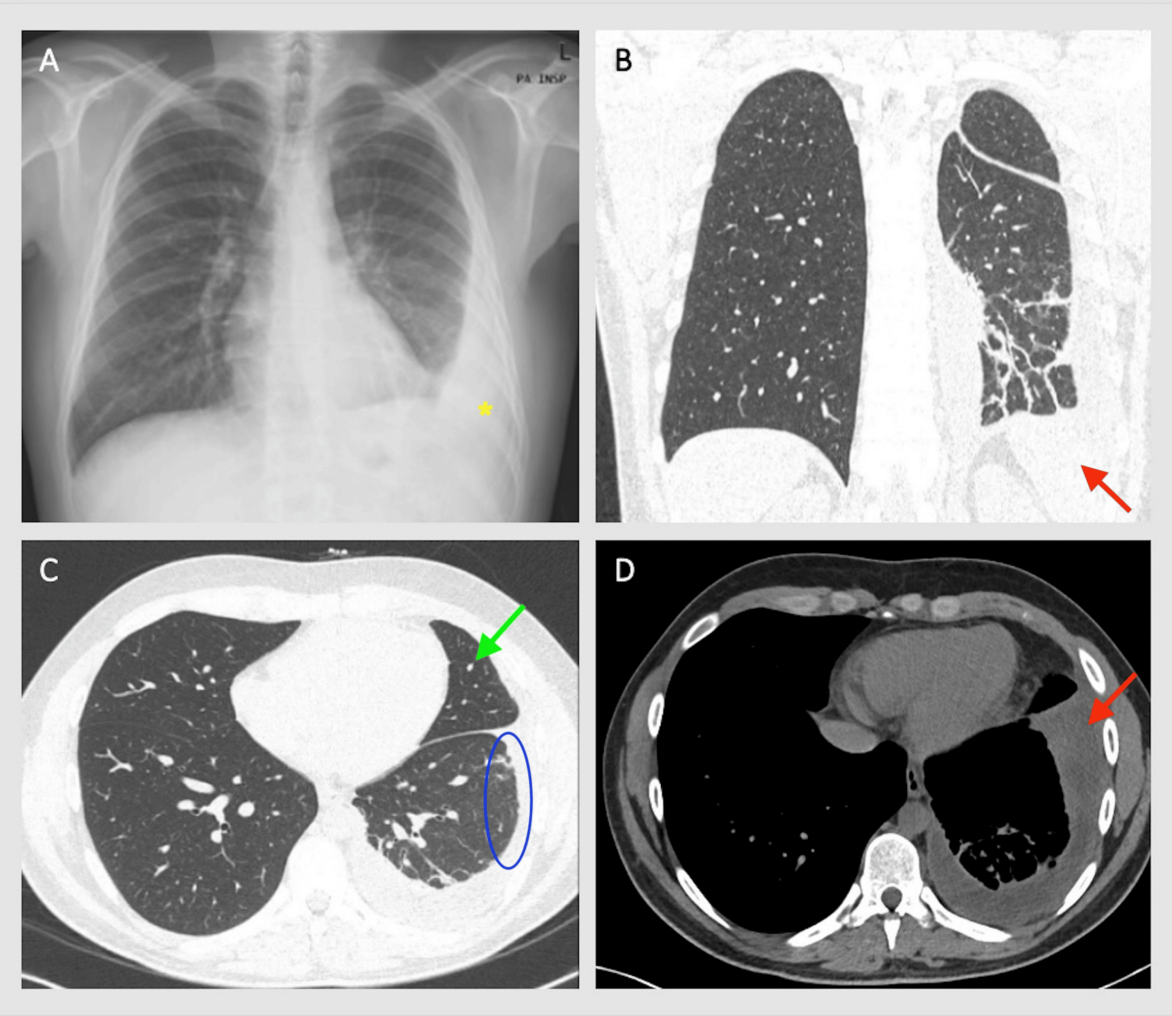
Chest radiography and chest HRCT images before initiation of glucocorticoid therapy. (A) Chest radiography showing left pleural effusion (yellow asterisk). (B) HRCT on the coronal plane reveals small-volume, organized, left-sided pleural effusion (red arrow). (C) HRCT on the axial plane demonstrating nodular pleural thickening (blue circle) and a 4 mm lingular nodule (green arrow). (D) HRCT on the axial plane showing organized left-sided pleural effusion (red arrow). HRCT: high-resolution computed tomography
